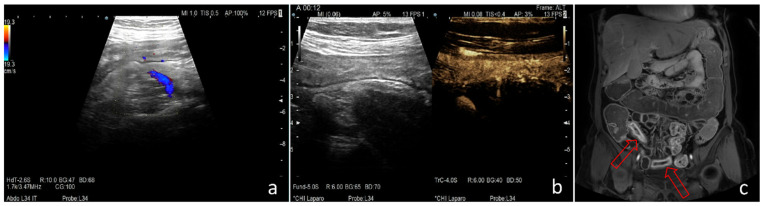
Comparison between the different scores for evaluating disease activity using IUS + CEUS or MRE in the case of a patient with a mild form of Crohn’s disease (CDAI = 152; HBI = 5; fecal calprotectin = 390 μg/g; CRP = 1.42 mg/L). (a) Colour Doppler imaging shows intermittent increases in vascularity—Limberg score = 2. (b) CEUS highlights transmural enhancement (Peak Enhancement = 38.86 dB, Wash-in Rate = 1750.75 [a.u], after the quantitative analysis of vascular pattern using VueBox^®^)—Ripollés score = 72.31, Medellin-Kowalewski score = 38.86. (c) MRE—T1 postcontrast phase (venous phase), coronal plane: the arrows indicate an increased wall thickness affecting the terminal ileum continuously, mainly with a mucosal contrast enhancement pattern in this phase and engorged vasa recta in the affected region—MaRIAs score = 3. In this case, we notice a discrepancy between the results of the imaging tests that suggest a severe form of CD and the results of the clinical activity scores and laboratory tests.
